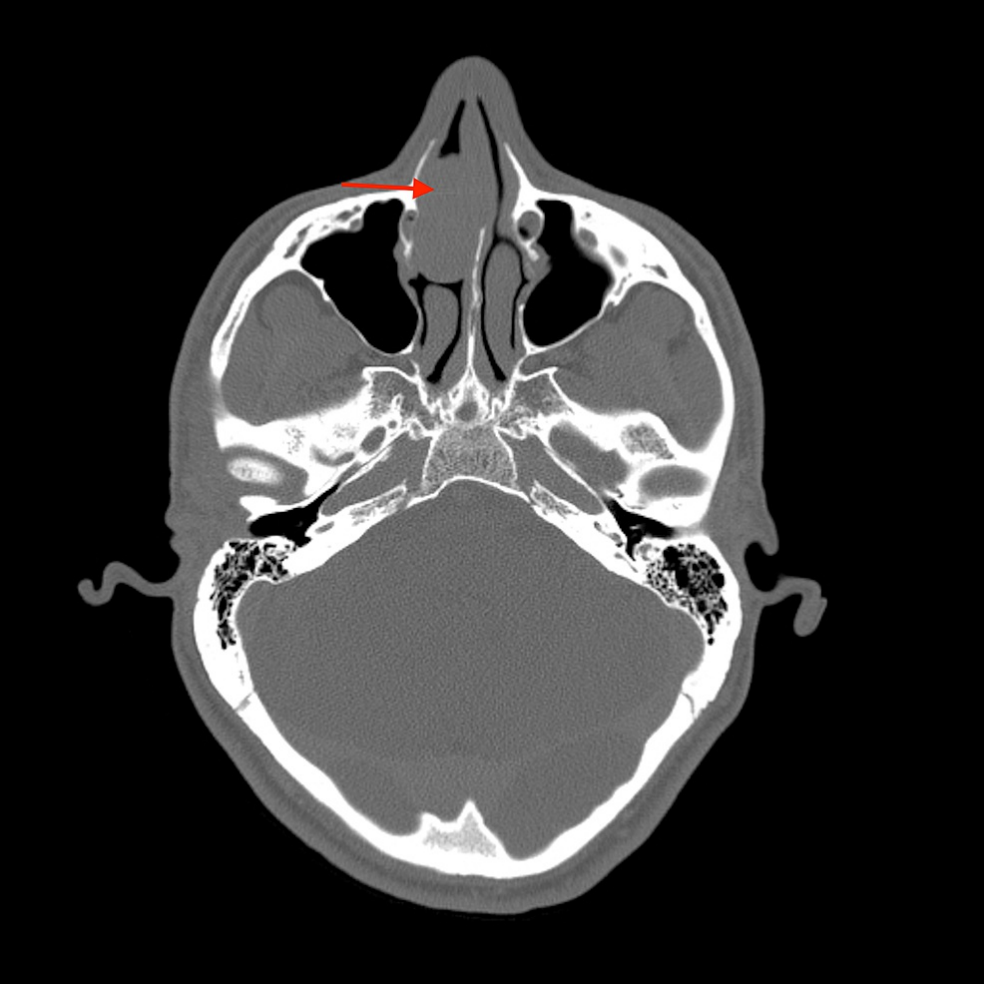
Axial soft tissue contrast-enhanced computed tomography scan of the paranasal sinuses and orbit
